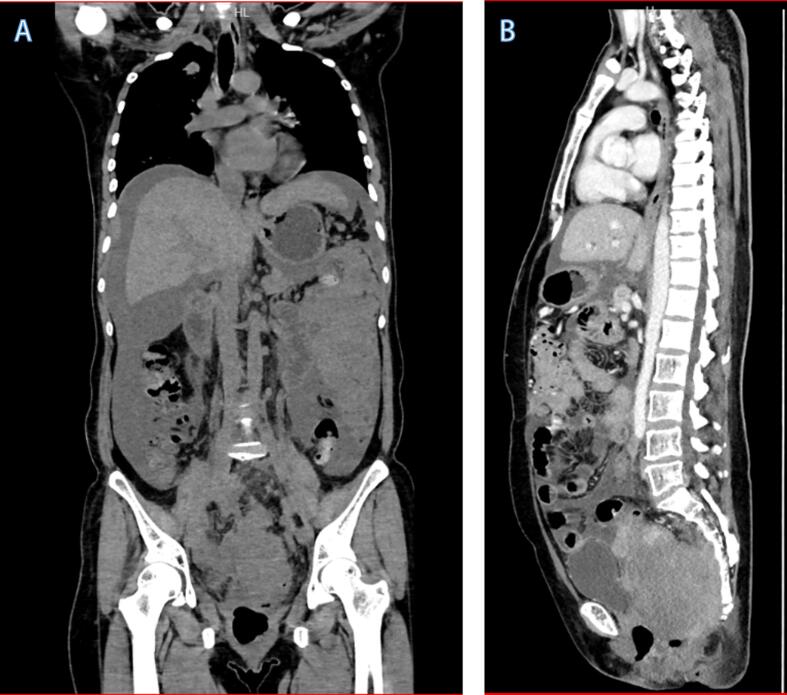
CT (A and B) showing multiorgan metastases after treatment.
